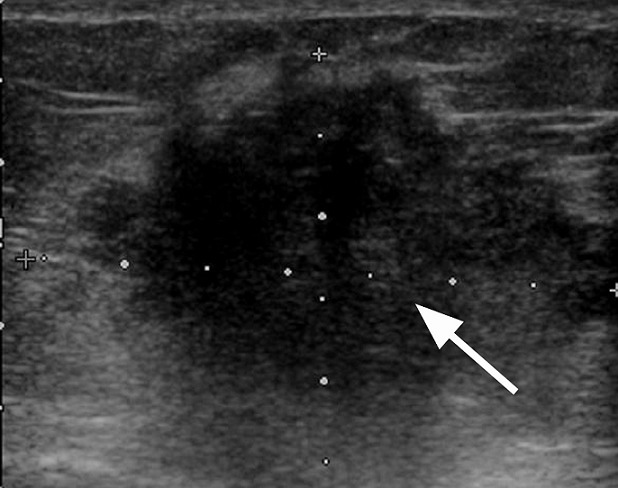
Ultrasound imaging confirms the microlobulated mass with posterior shadowing in the right breast revealing malignant characteristics (arrowed)
